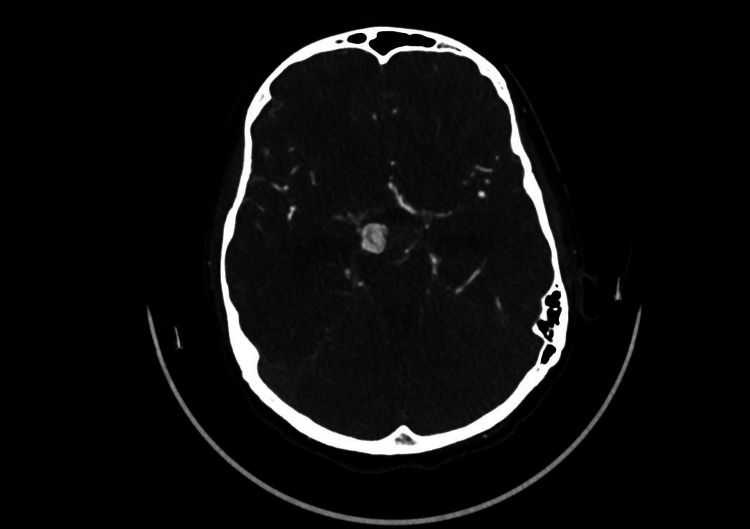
CT cerebral angiography (axial view) demonstrating a well-circumscribed saccular aneurysm arising from the right posterior communicating artery, clearly visualized within the circle of Willis
